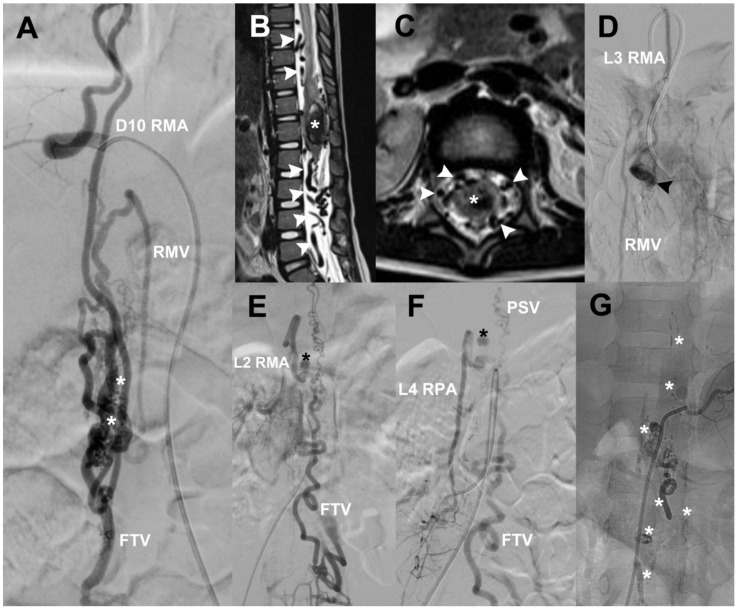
(A) A diffuse CM sAVM case; white asterisks mark the place of the diffuse nidus, and the first afferent is right D12 RMA while the drainage goes to RMV and FTV. Lumbosacral T2-MRI shows flow voids (white arrowheads) and hematomyelia (white asterisks) (B,C). (D) One fistulous component at left L3 RMA; a venous aneurysm (black arrowhead) and its drainage through RMV. Other fistulas are seen at right L2 RMA (E) and right L4 RPA (F) while their drainage goes through PSV and FTV; a small arterial aneurysm (black asterisk) can be seen. (G) A negative control sDSA after Histoacryl embolization (white asterisks) and surgical management. CM = conus medullaris, RMA = radiculomedullary artery, RPA = radiculopial artery, RMV = radiculomedullary vein, FTV = filum terminale vein, PSV = posterior spinal vein.
